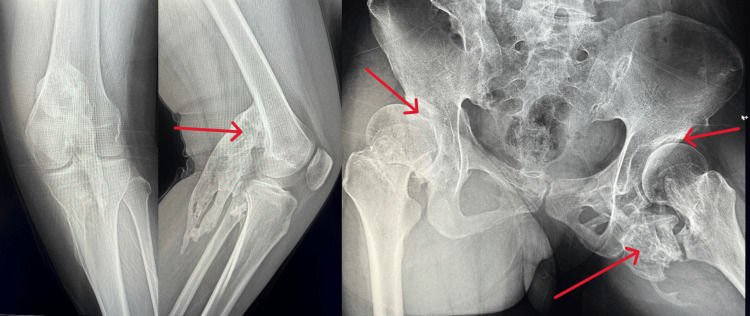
The X-ray of the left knee and hip There was a large mass in the left knee joint, and dysplasia and multiple large masses in the hip joint.
